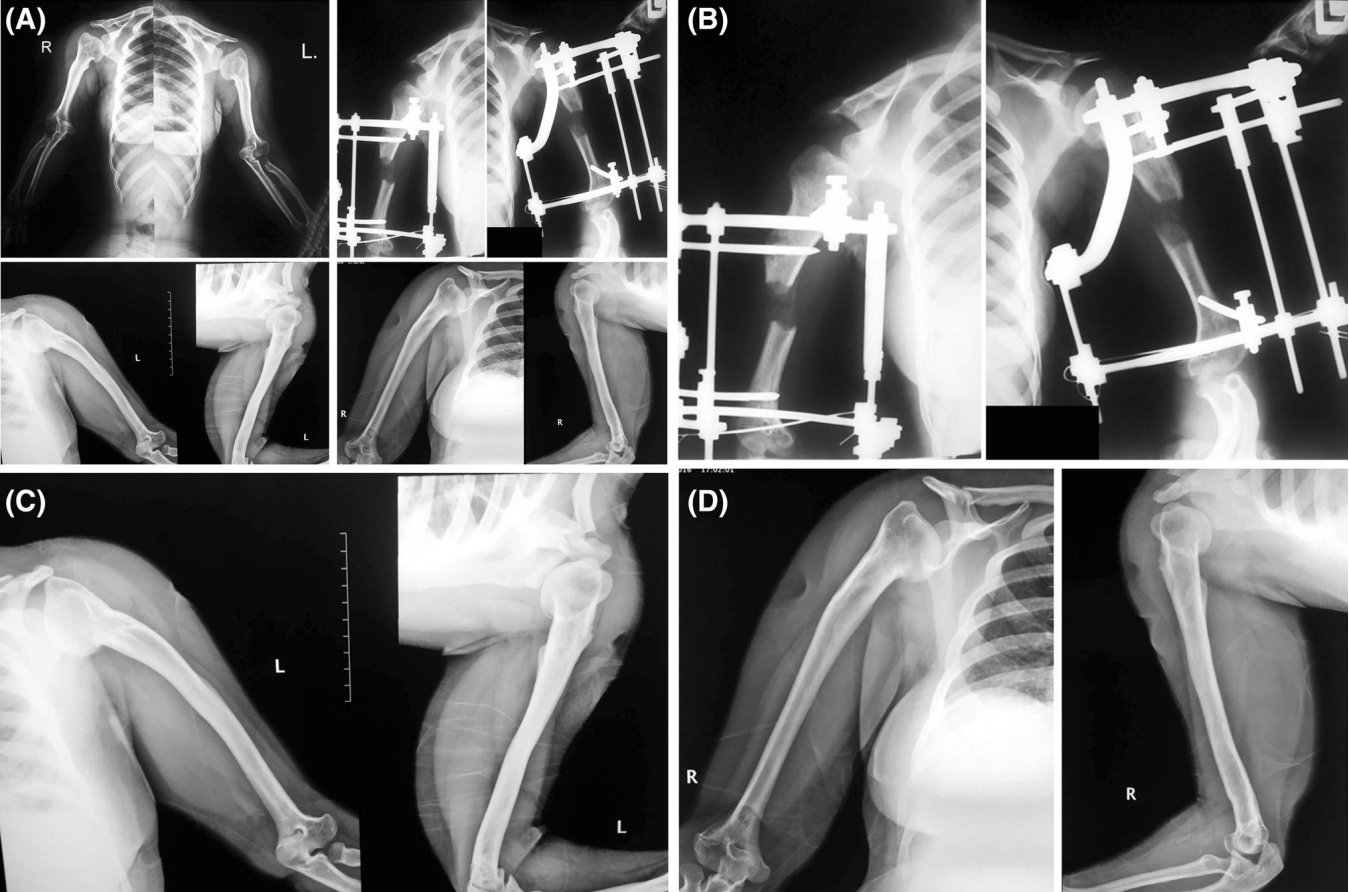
a Anteroposterior radiograph of both humeri in a 17-year-old girl with achondroplasia. b X-ray during lengthening. c Anteroposterior and lateral X-rays after 7-year follow-up. d Anteroposterior and lateral X-rays after 7-year follow-up
